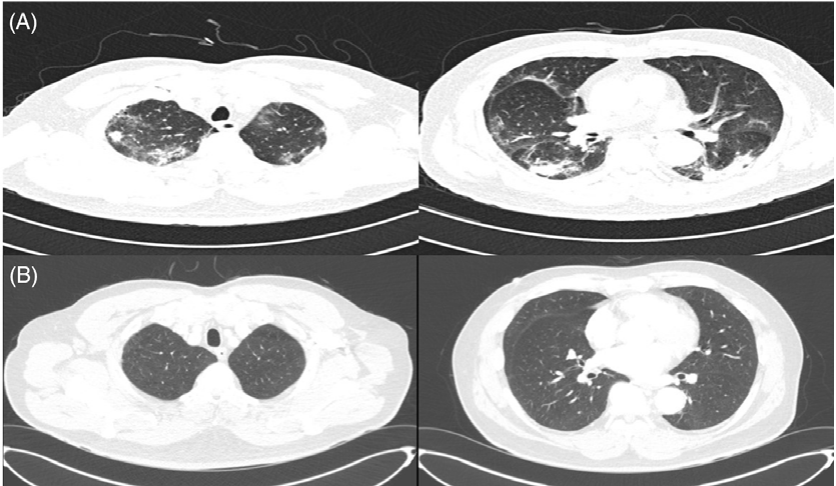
(A) Chest CT on illness day 20 and hospitalization day 18 (March 18). (B) Chest CT at the 3-Mo follow-up (July 4).
